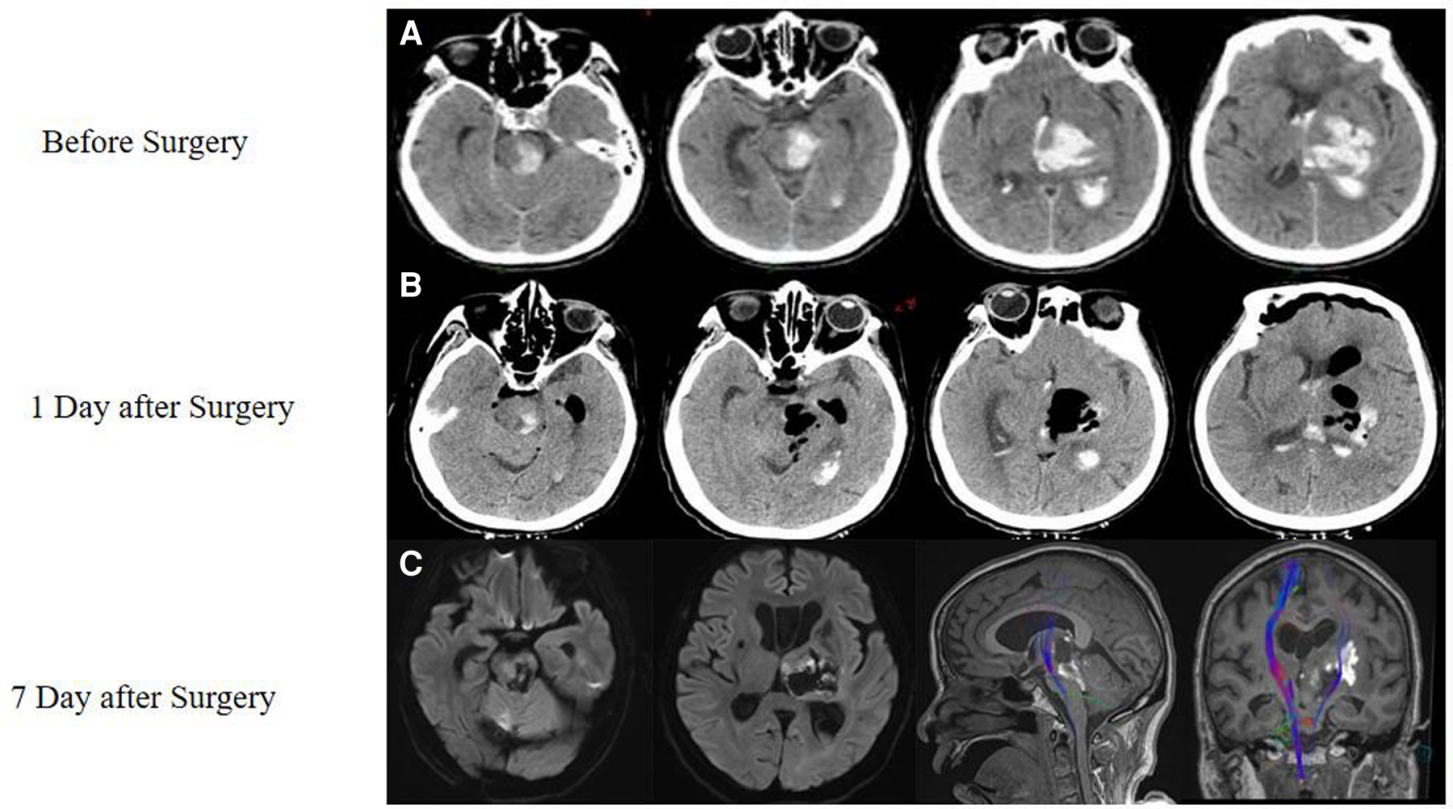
Case of a thalamic hemorrhage with extension to the brainstem. (A) An initial CT scan performed in the emergency room showed thalamic hemorrhage with extension to the brainstem and displayed a hematoma from the midbrain to the thalamus. (B) On the first day after the surgery, another CT showed that most of the hematoma had been removed. (C) Seven days later, a magnetic resonance imaging (MRI) scan was performed, which showed that a part of the white fiber had been saved, as shown by DTI.
